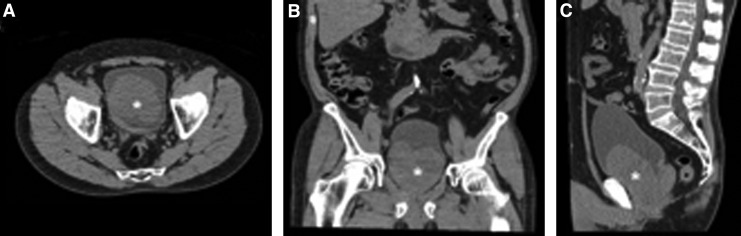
CT scan of the 10cm x 9cm x11cm prostate gland (*) in axial (A), coronal (B) and sagittal (C) views demonstrating the large intravesical component.
